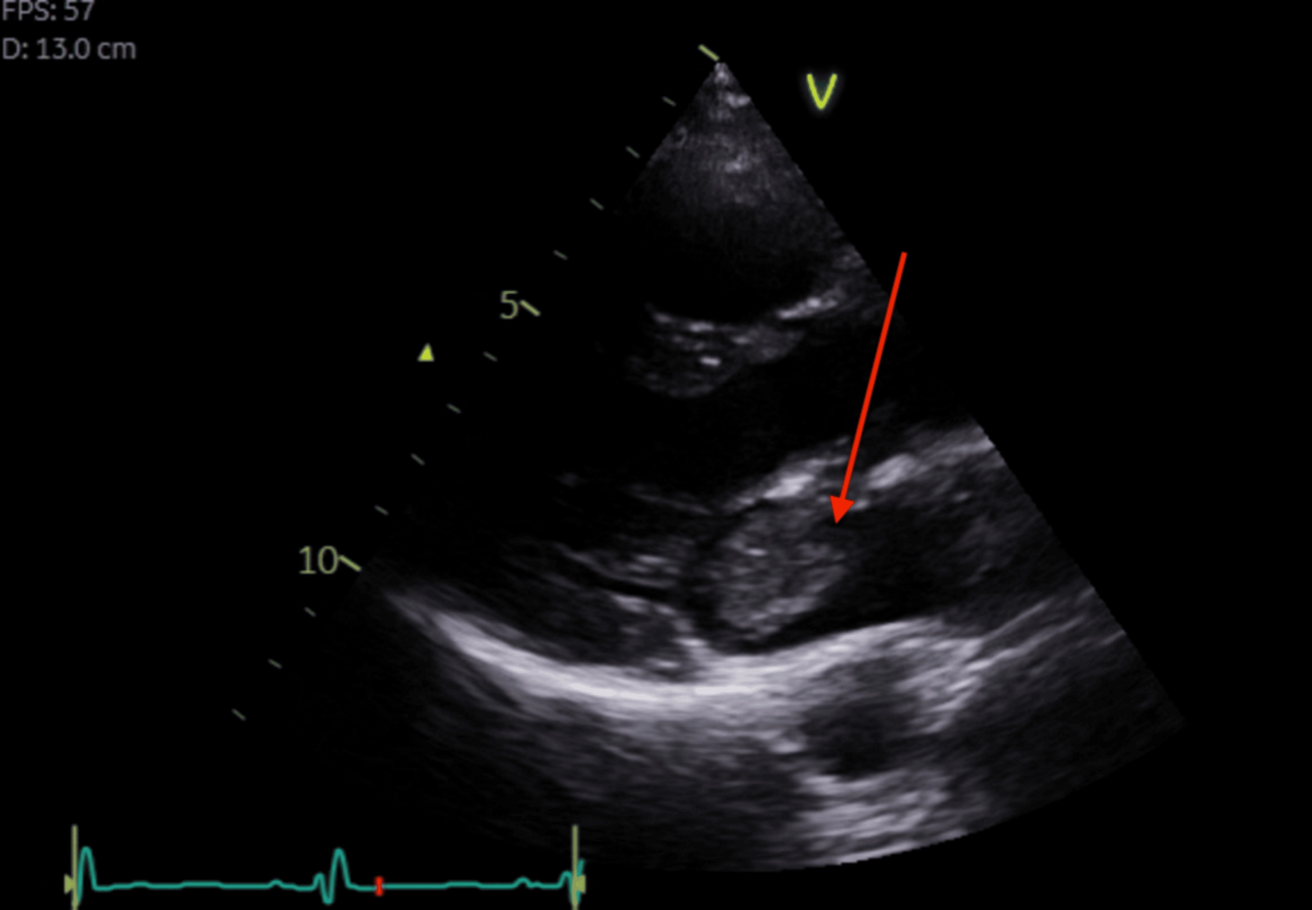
Transthoracic echocardiogram showing a mobile echogenic mass measuring 29 mm × 15 mm (red arrow), consistent with a left atrial myxoma.
